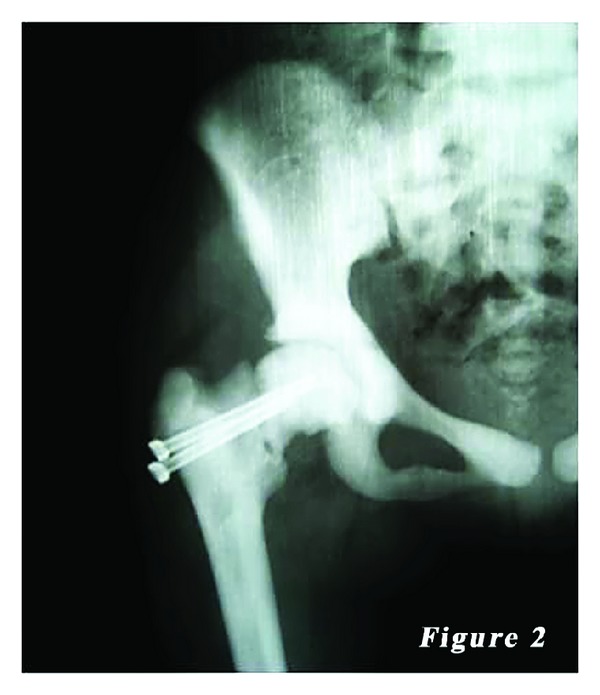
Postoperative radiography of the first patient.
